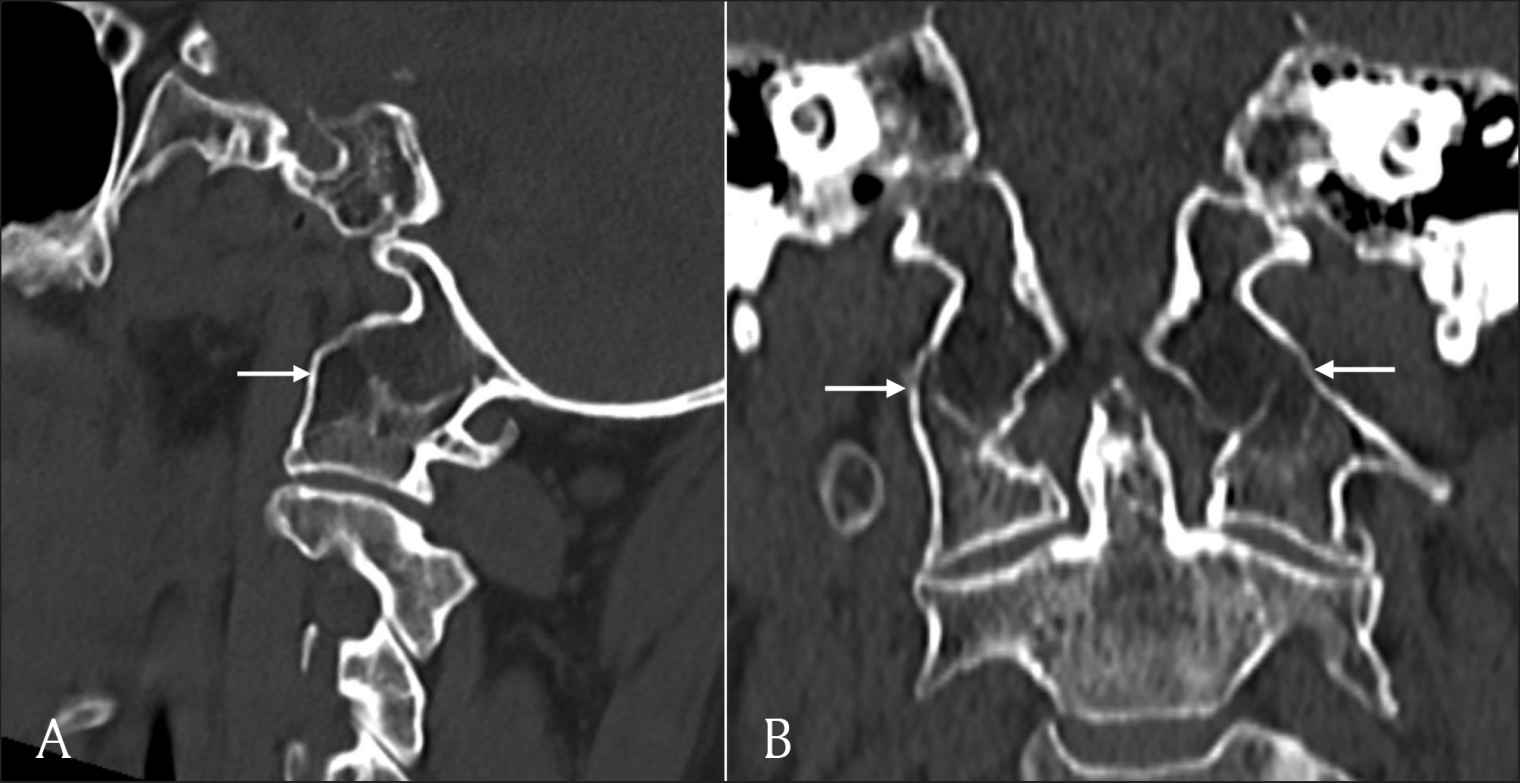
Sagittal (A) and coronal (B) reconstructions of a CT scan of the cervical spine shows assimilation of the massa lateralis of C1 and the occipital condyle (white arrows).
